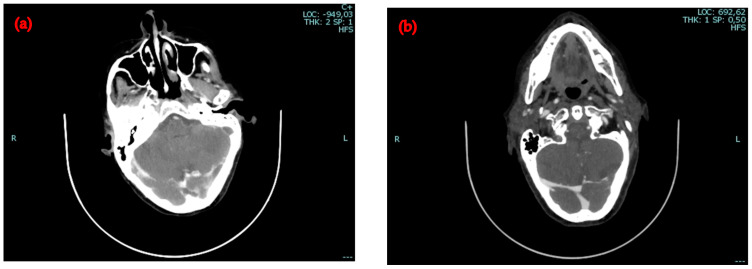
Comparison between brain CT scans with contrast on admission and discharge: (a) complete thrombosis of the left transverse sinus and partial thrombosis of the right transverse sinus; (b) resolution of the partial thrombosis of the right transverse sinus, while on the left side, the thrombo-embolic opacification defect persists, affecting the transverse sinus, the sigmoid sinus, and the proximal portion of the internal jugular vein.
